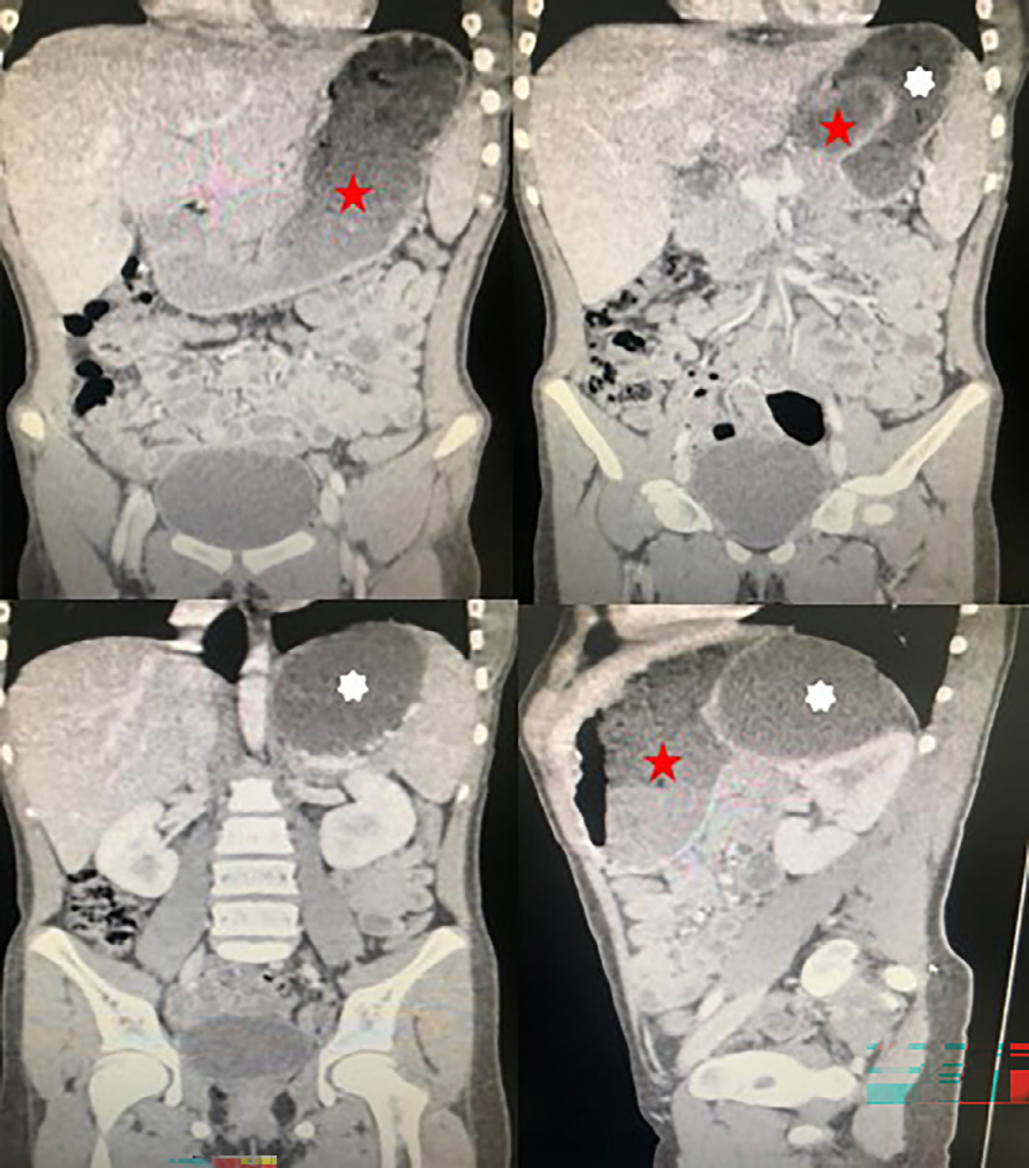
Injected abdomino-pelvic CT images in coronal (A-C ) and sagittal (D) reconstruction showing a non-communicating retro-gastric cystic formation, measuring approximately 87 × 59 mm (White Astérix). Red Star (Stomach).
